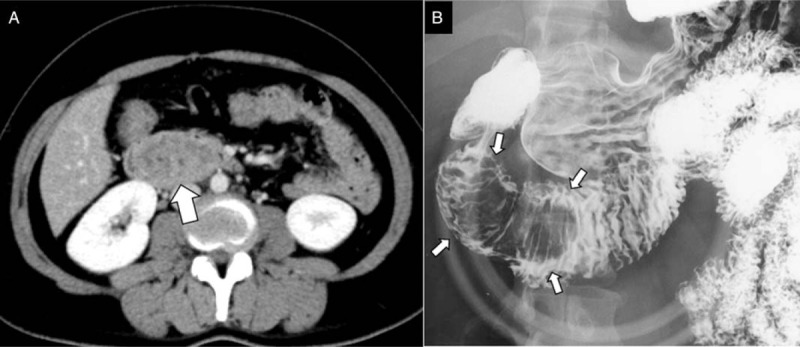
CT analysis (A) and barium x-ray examination (B). A mass measuring about 55 mm in size was shown near the proximal duodenum. (White arrows). CT = computed tomography.
